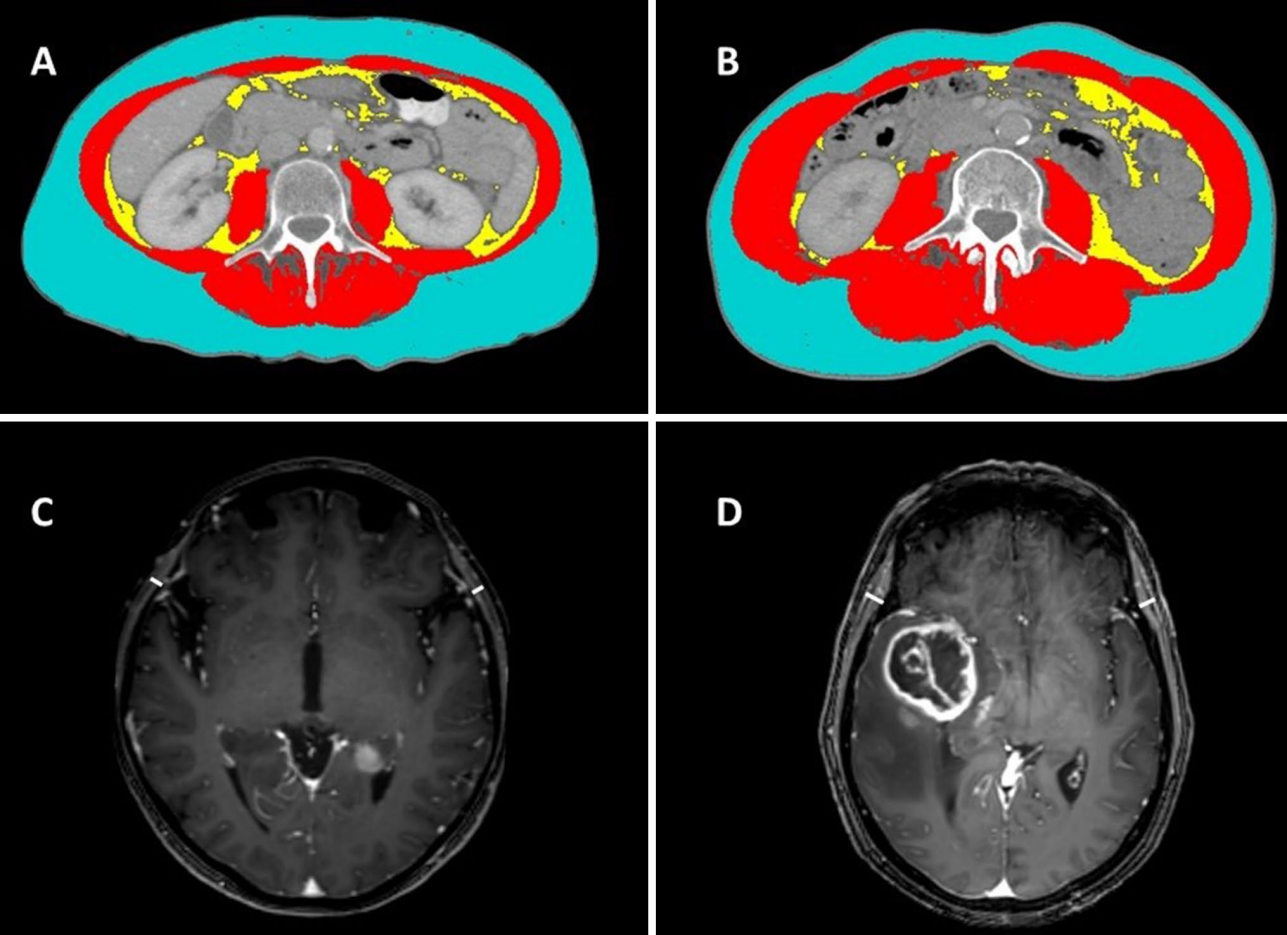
Examples of SMA assessment on transverse abdominal CT slices (A, B) and TMT assessment on axial T1-weighted contrast enhanced cranial MR images (C, D). A and C A 53 year old female at risk of sarcopenia according to TMT assessment (SMA = 76 cm^2^; TMT = 3.4 mm). B and D A 63 year old female with normal muscle status according to TMT assessment (SMA = 140 cm^2^; TMT = 8.3 mm). Red: skeletal muscle; Yellow: visceral fat; Blue: subcutaneous fat
